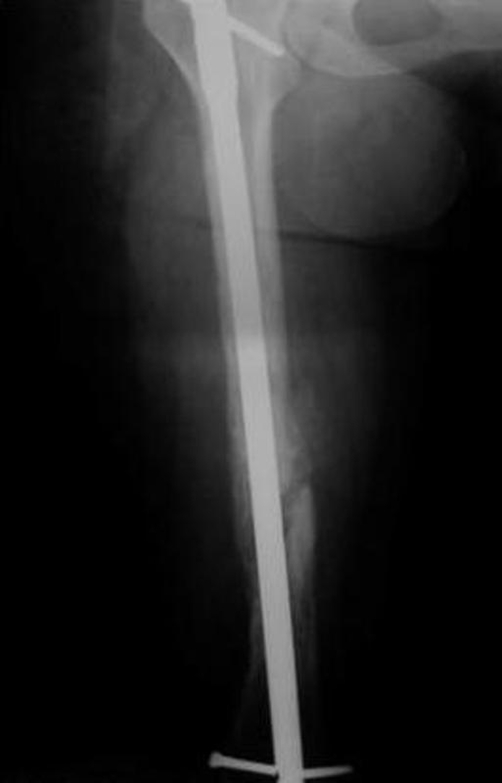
Post-operative radiograph showing union at the fracture site.
